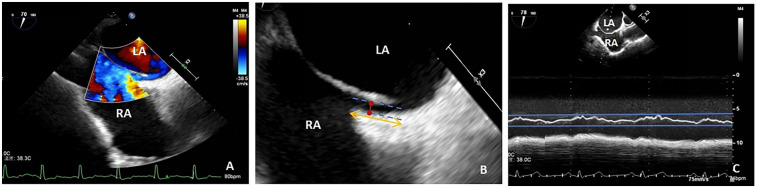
(A) TTE shows a diagonal right-to-left shunt between the left and right atria, diagnosed as patent foramen ovale (PFO); (B) PFO width, maximum distance between the primary and secondary septa (red arrow); PFO tunnel length, length of overlap between the septum primum and the secundum (yellow arrow); (C) atrial septal aneurysm, M-mode measurement of septum primum excursion ≥ 10 mm from the plane of the atrial septum into the right atrium; LA, left atrium; RA, right atrium; TEE, transesophageal echocardiography.
